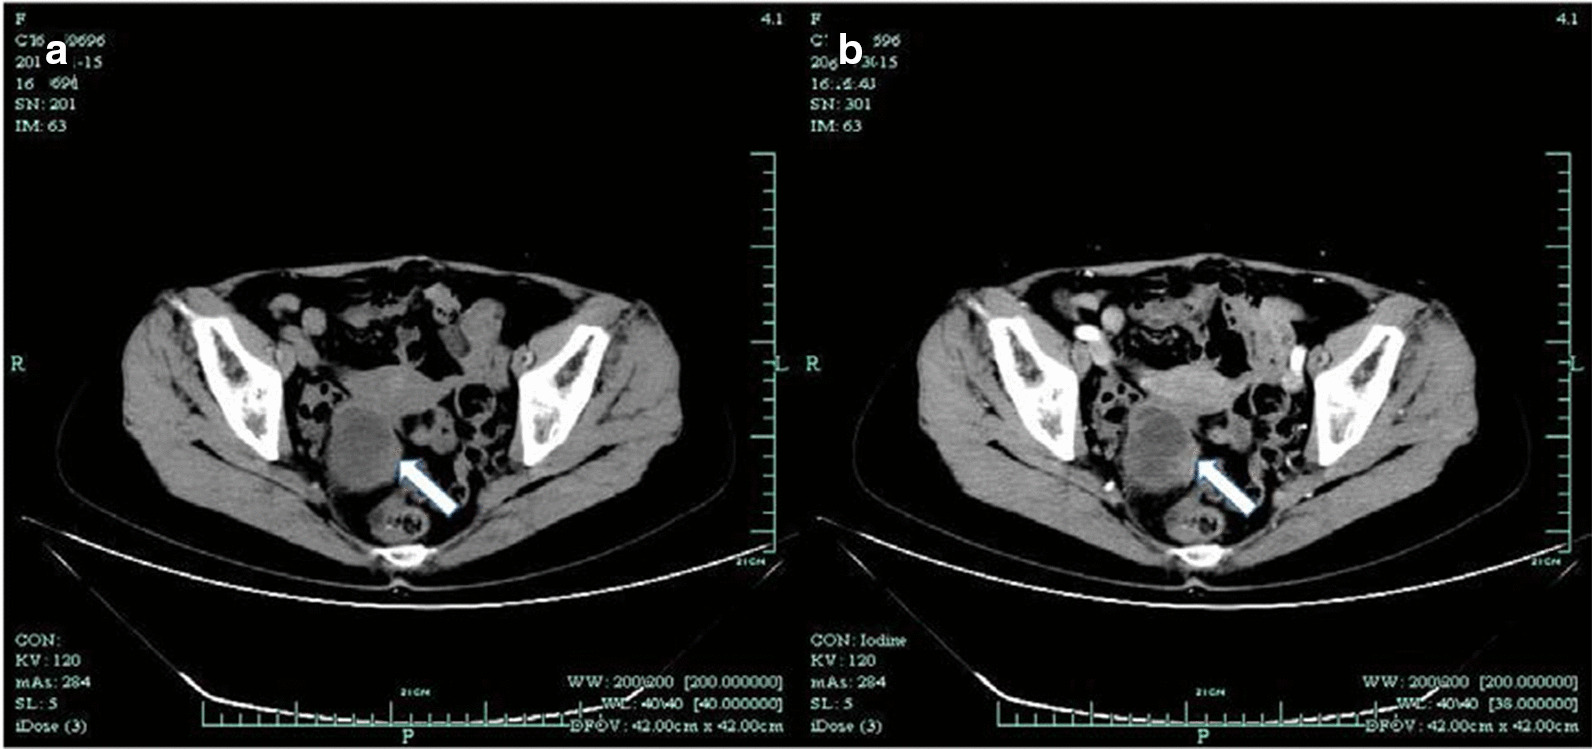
a Axial CT image showed s a well-defined heterogeneous mass with central hypodense areas in the right retroperitoneal space (arrow). b Contrast-enhanced CT scan showed hyperattenuating of papillary excrescence of the mass (arrow)
